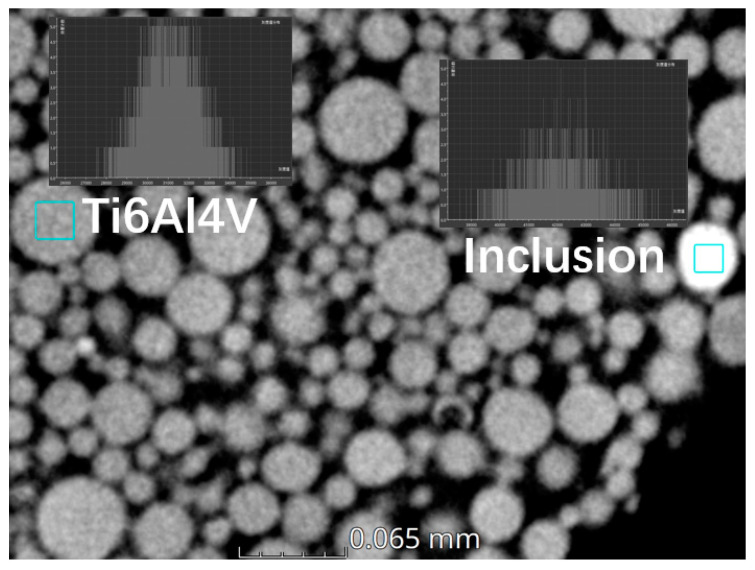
The gray value of Ti6Al4V particles and inclusions in the CT image.
